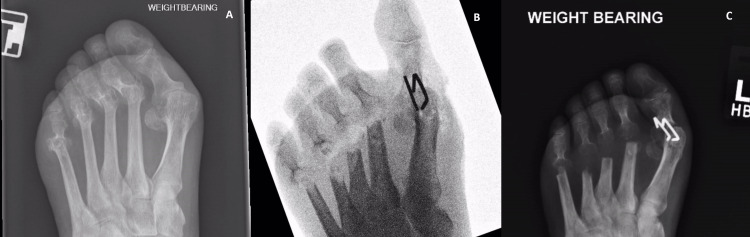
Serial X-rays of the left foot of a patient who underwent the first MTPJ fusion A: Preoperative X-ray; B: Intraoperative X-ray; C: Postoperative X-ray; MTPJ: Metatarsophalangeal joint
